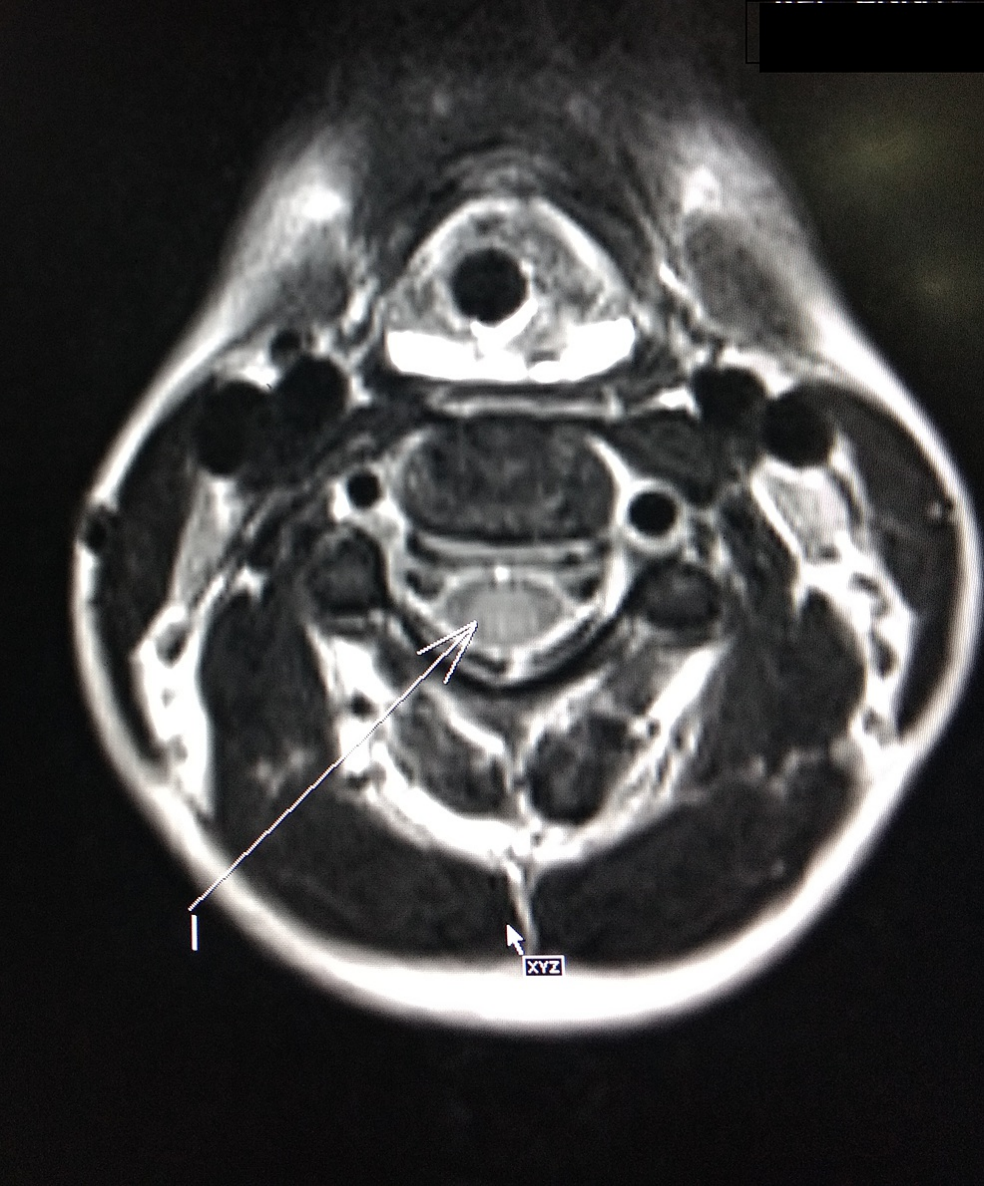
Axial section MRI cervical spine showing intramedullary T2W hyperintensity Note that on the axial image it is seen predominantly involving the central region, relatively sparing the lateral aspect MRI: magnetic resonance imaging
